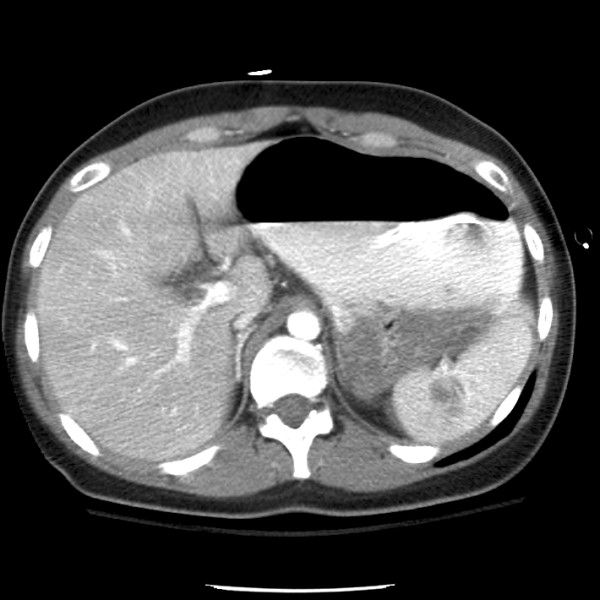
Abdominal CT scan of parenchymal splenic laceration without obvious vascular injury at 01:16 am.
